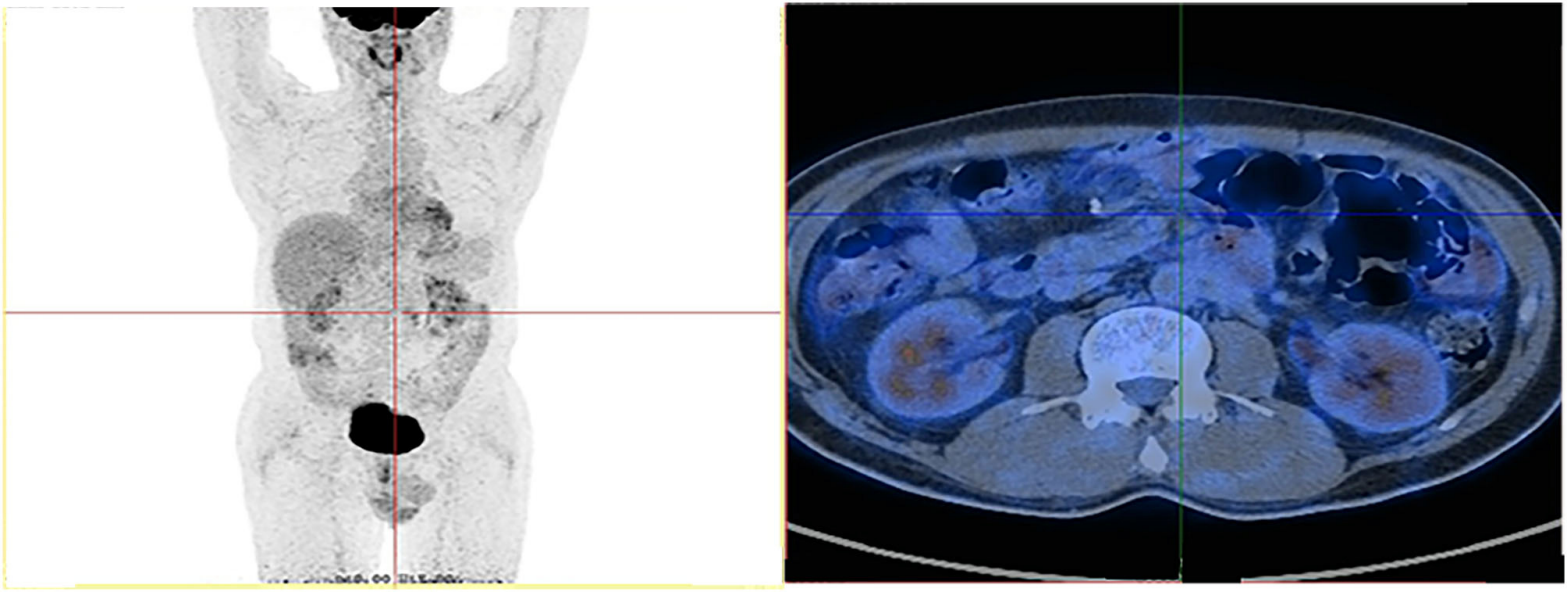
FDG-PET scan shows no metastatic lesions and lymph node.
